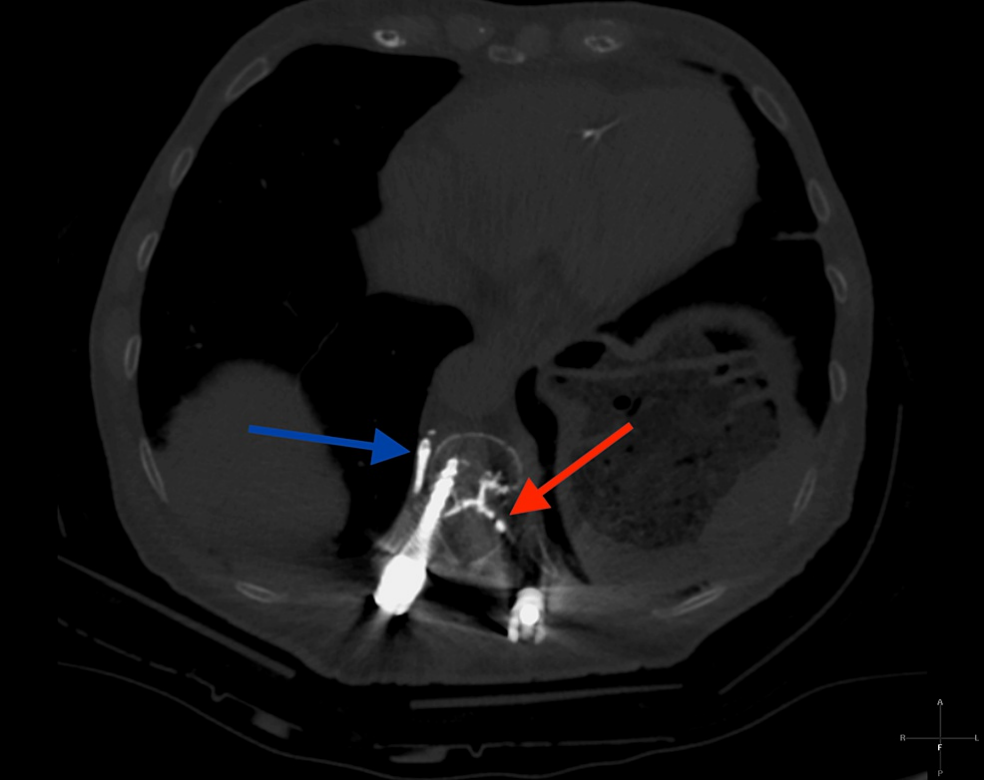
CT imaging of previous cement extravasation An axial CT image of the chest demonstrates extravasated cement from the prior spinal fusion surgery. This is seen in a paravertebral vein (blue arrow) and along Batson’s plexus extending to the epidural space (red arrow).
